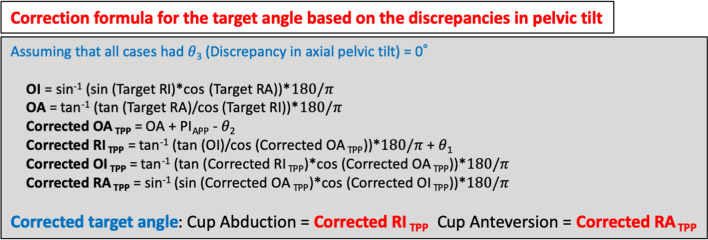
Correction formula for the target angle based on the discrepancies in pelvic tilt, as measured by TPP images. In the future, we plan to use this correction formula to determine the target cup orientation angle. It will be necessary to minimize the angle of θ_3_ in order to achieve more accurate installation. RI, radiographic inclination; RA, radiographic anteversion; OI, operative inclination; OA, operative anteversion; TPP, table parallel plane; RI_TPP_, RI based on the TPP; RA_TPP_, RA based on the TPP; OI_TPP_, OI based on the TPP; OA_TPP_, OA based one TPP; θ_1_, discrepancy in coronal pelvic tilt; θ_2_, discrepancy in sagittal pelvic tilt; θ_3_, discrepancy in axial pelvic tilt; PI_APP_, pelvic inclination of anatomic pelvic plane in the supine position.
